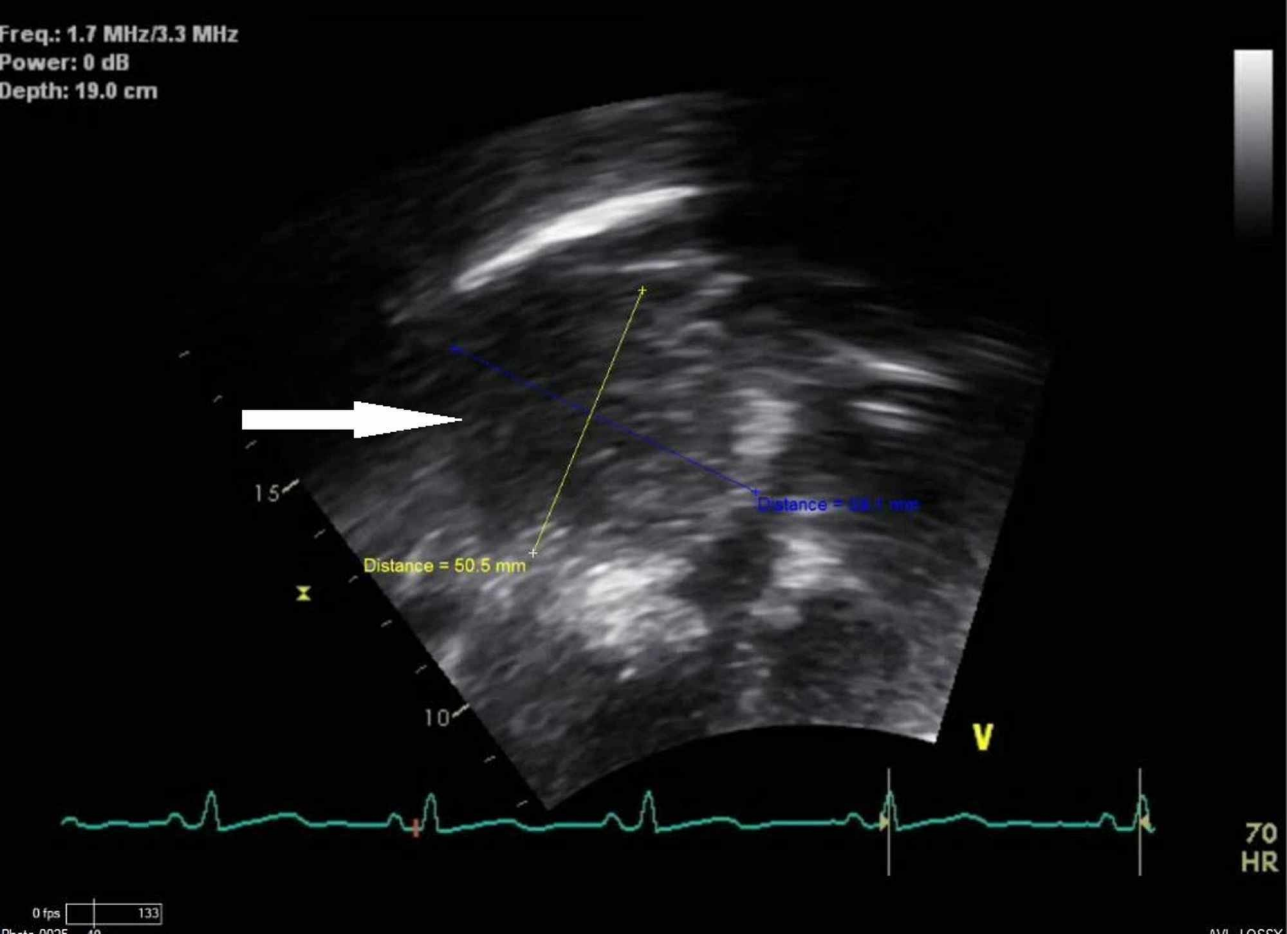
Transthoracic echocardiogram apical four-chamber view still image with homogenous appearing, circular, sessile mass in front of the right atrium (white arrow) measuring 6.1 cm X 8.7 cm in dimension with evidence of right atrial compression
